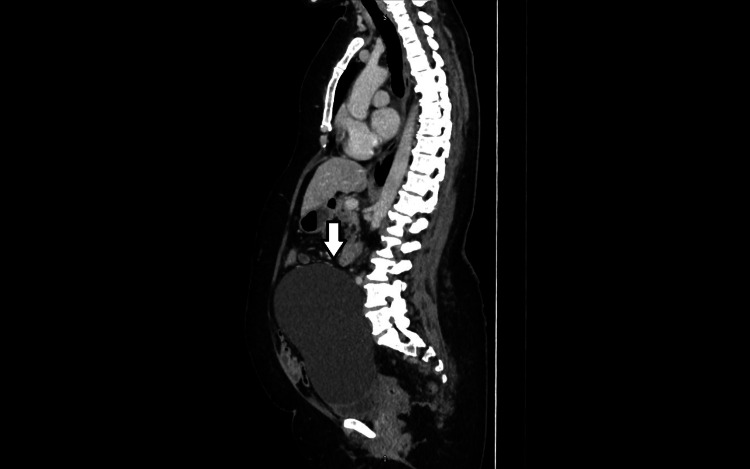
Sagittal CT image of the same cystic lesion adjacent to the sigmoid colon, which was confirmed intra-operatively to arise from the sigmoid mesentery. Cross-sectional imaging demonstrated a large cystic lesion measuring approximately 19 cm in maximum dimension
